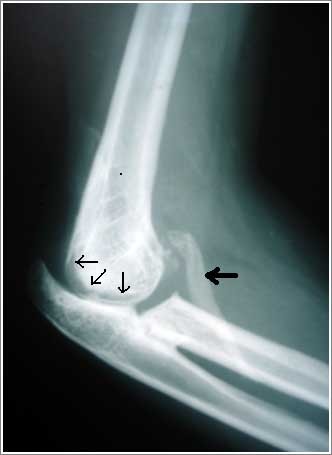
Postoperative lateral radiograph of 28 year female showing the well formed elbow joint(marked by thin black arrows) with unexcised static HO anteriorly (shown by thick black arrow) at 8 months follow up.
